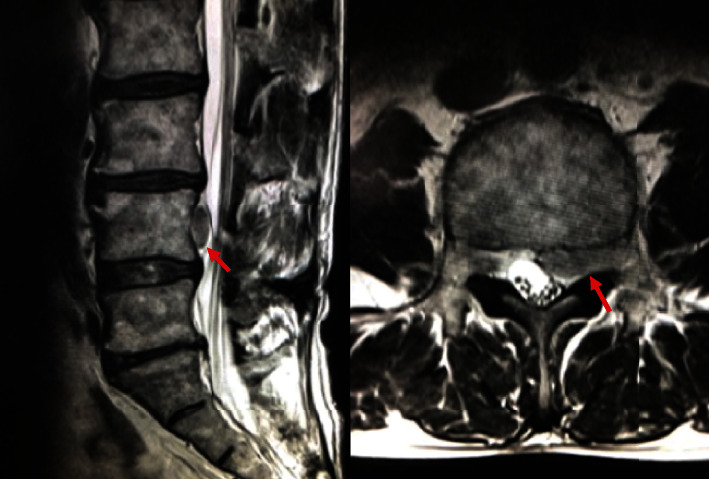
Postdiscogram MR images showed herniated fragment migrated to cranial central canal which was not detected in previous images.
